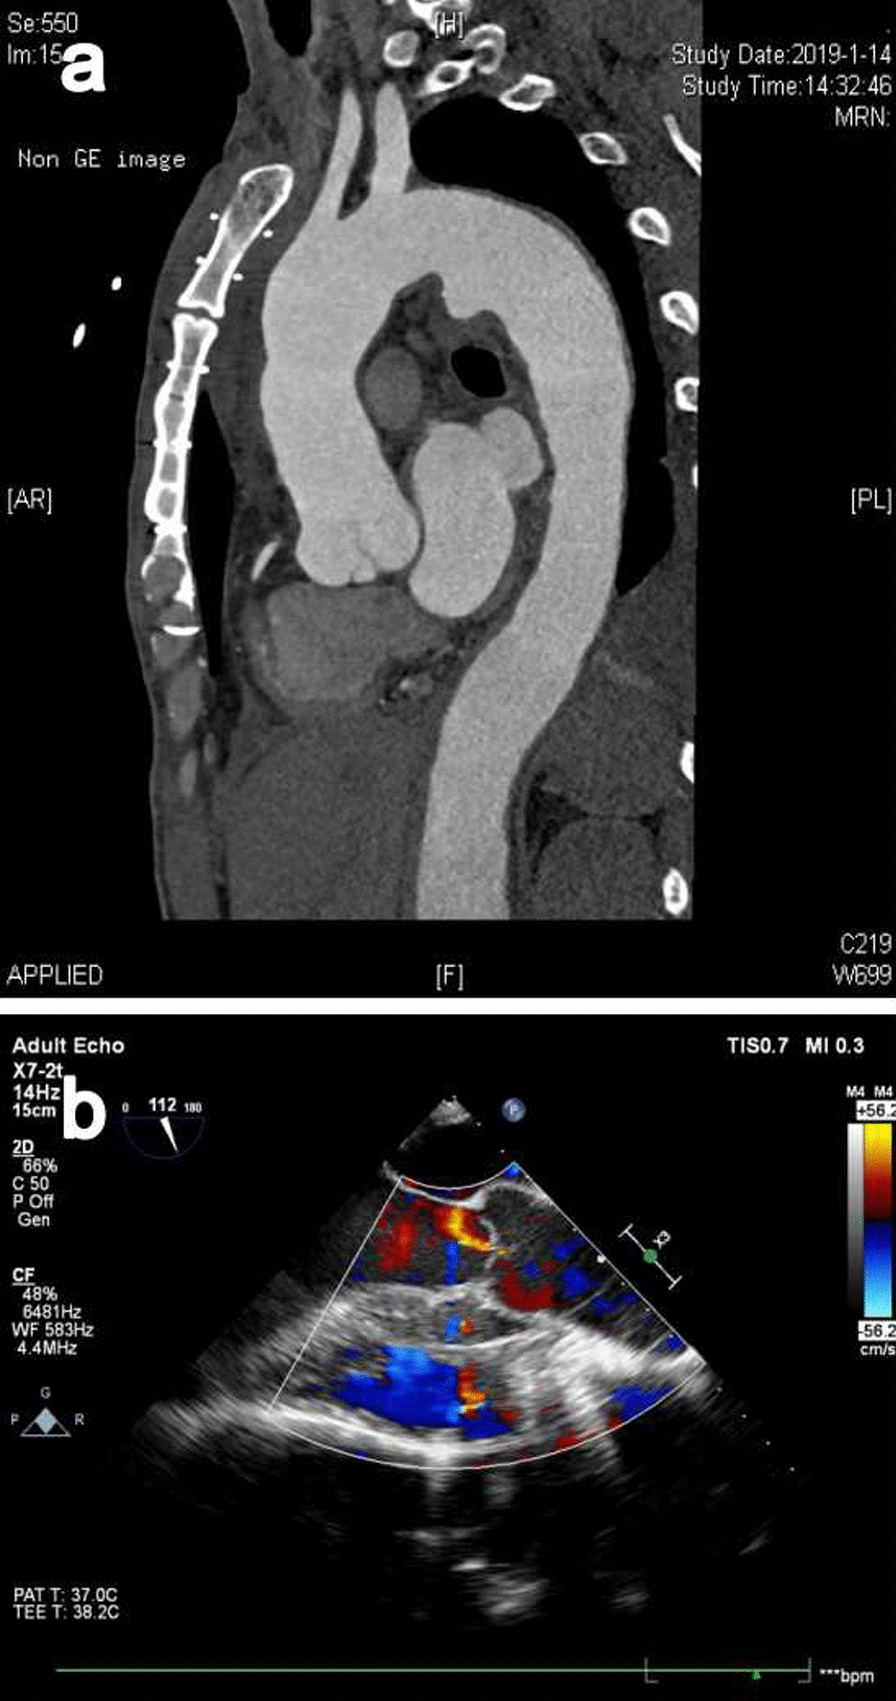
Postoperative examination. Aortic CT-scan (a) and Transthoracic echocardiography (b) image were performed and demonstrated no residual ALVT, and no aortic regurgitation
